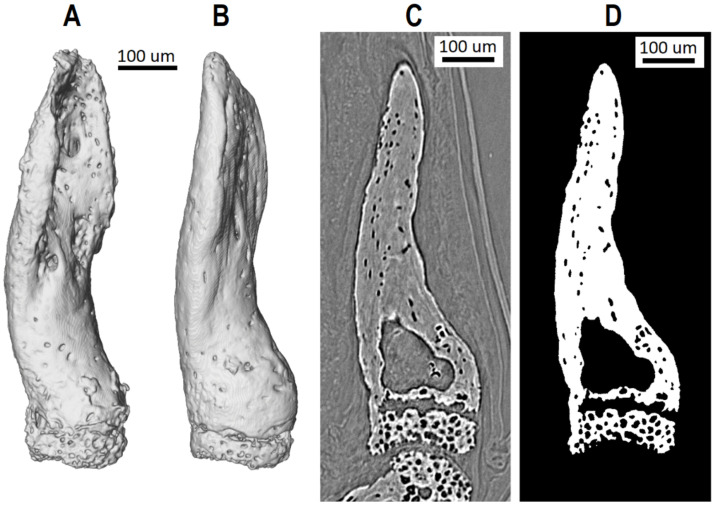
X-ray phase-contrast tomography of the 4th phalanges of gecko’s digits. (A,B)—the three-dimensional images of samples N°21 and N°18; (C)—the longitudinal tomographic sections (N°18); (D)—the segmented bone tissue (N°18).
